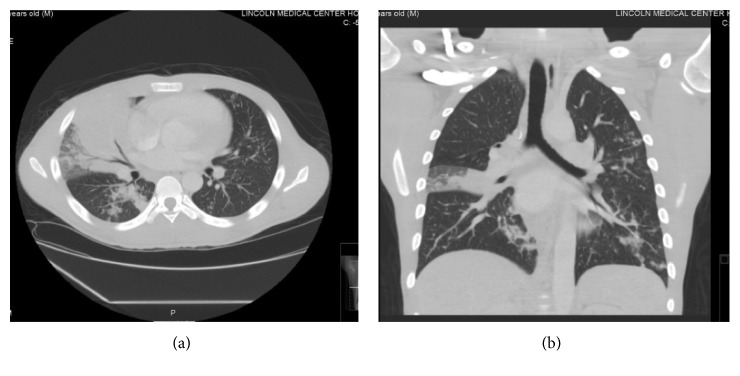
(a) CT chest (axial view) on the day of admission showing the right middle lobe consolidation with a possible necrotic medial segment and enlarged right hilar lymph nodes. (b) CT chest (coronal view) on the day of admission.
